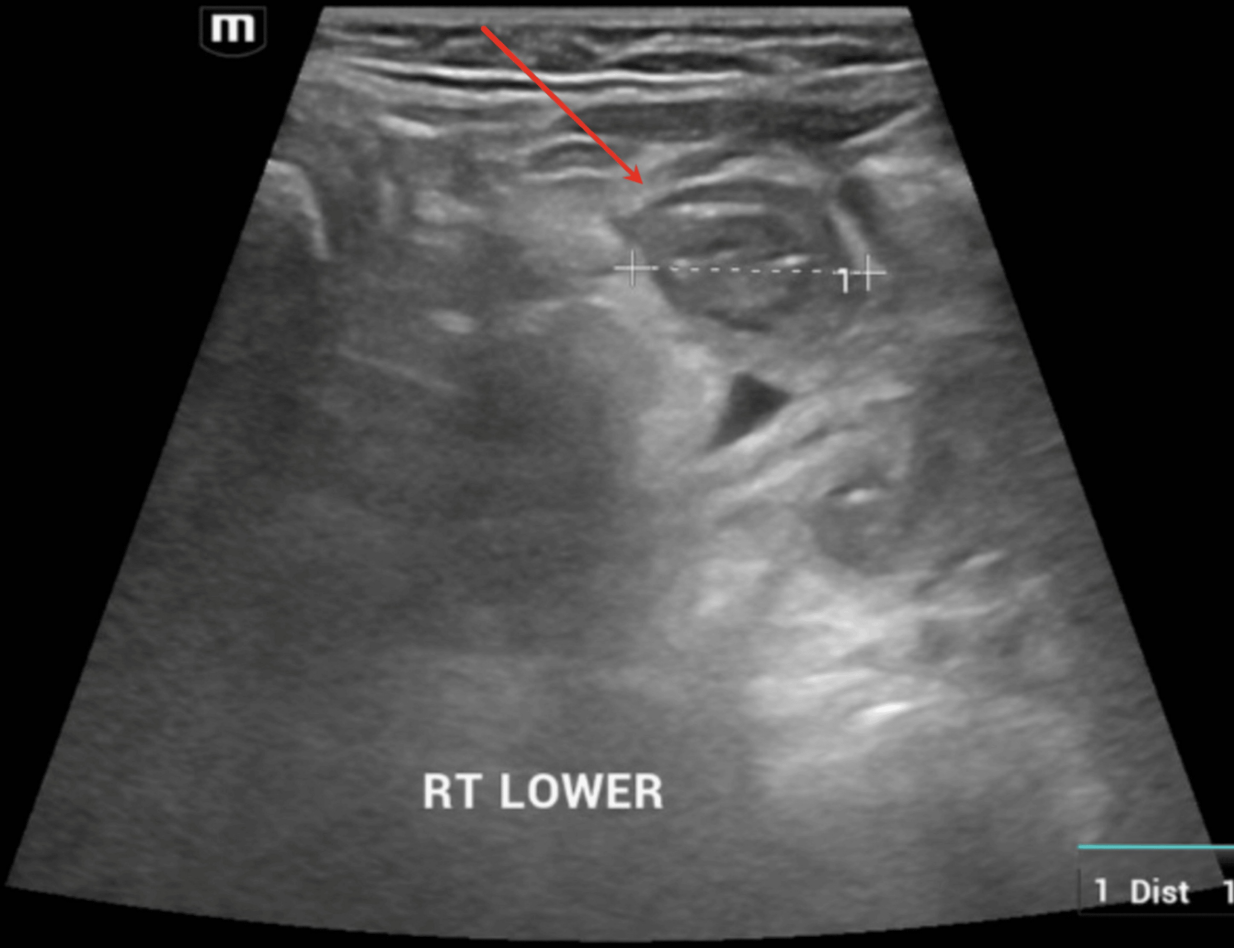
Ultrasound abdomen transverse view showing a doughnut sign Red arrow pointing at the doughnut sign
